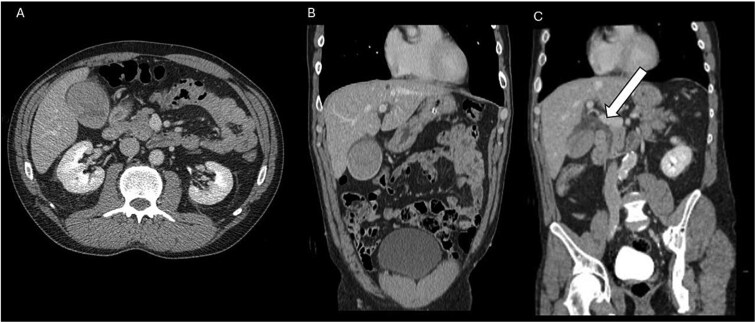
Follow-up CT scan. (A) Axial image showing the gallbladder lumen completely filled with hyperdense material. This finding is consistent with extensive endoluminal hemorrhage, likely compounded by the concurrent vicarious excretion of contrast medium from the previous administration, accompanied by the new appearance of pericholecystic fluid and mild thickening of the gallbladder walls. (B) Coronal reconstruction in the venous phase confirming the findings. (C) Coronal reconstruction in the venous phase revealing, in addition to the described findings, the new appearance of extrahepatic biliary tract dilation (arrow).
